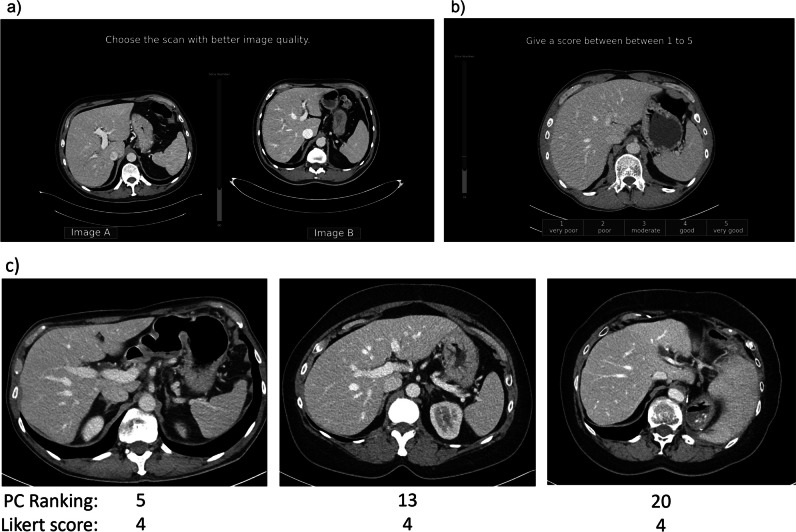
User interface of the in-house developed software for (a) the pairwise comparison method; b the Likert scale method. c Examples of 3 CT images ranked by an observer using the pairwise comparison method with corresponding Likert scores
